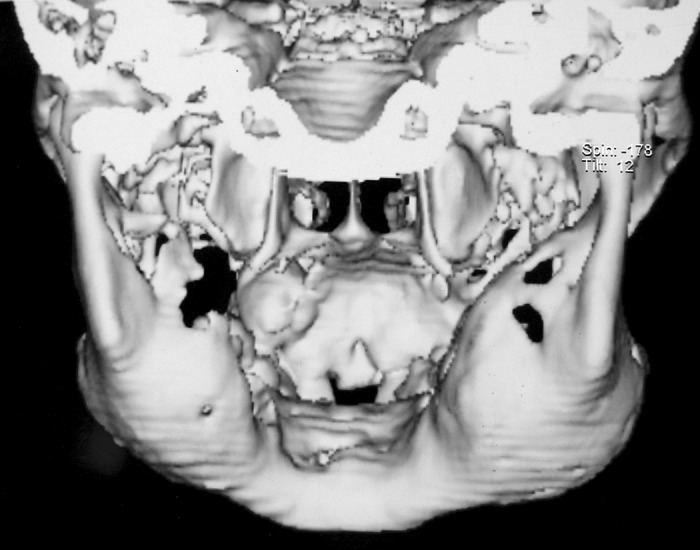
3D computed tomographic image of posterior view
